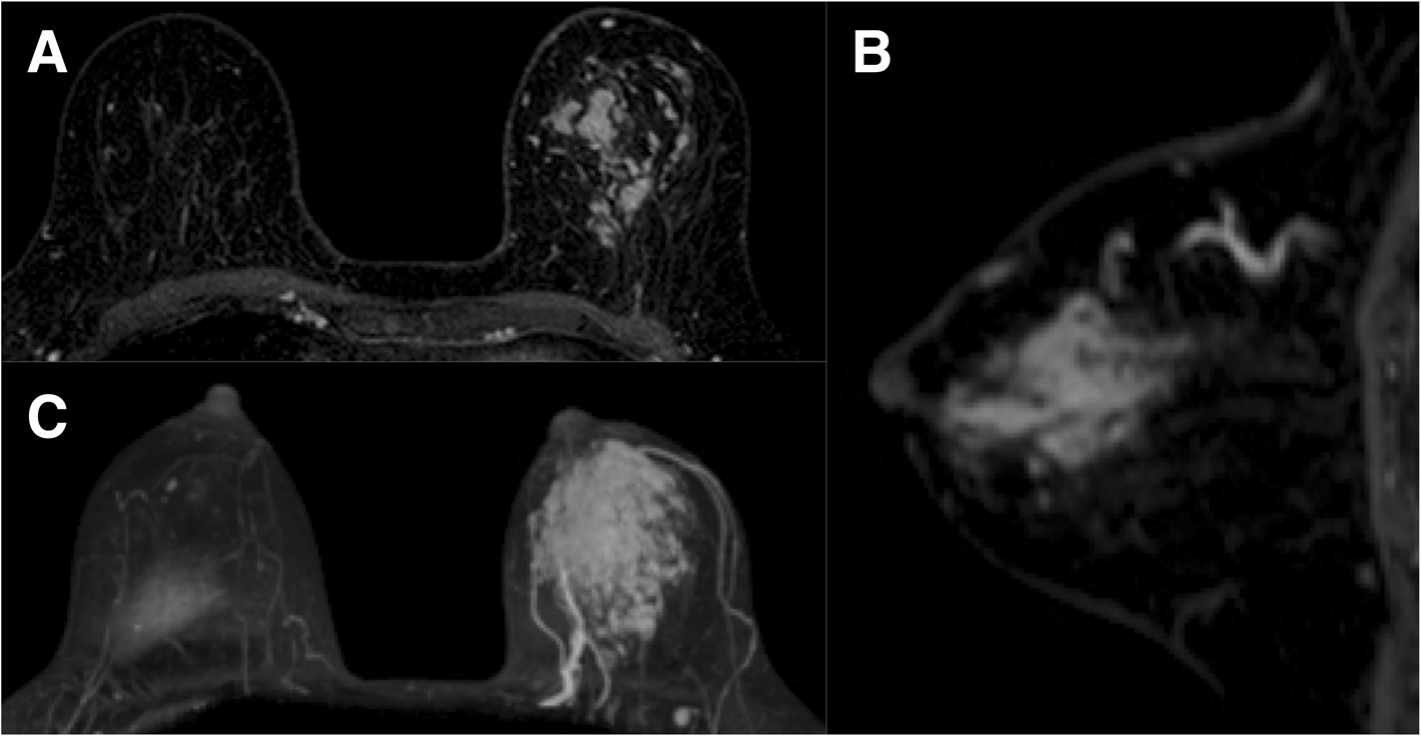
Findings from an enhanced magnetic resonance imaging scan of the breast. a, b Fat suppression. c MIP. Numerous punctate areas of contrast with dynamic washout were detected throughout the left breast. No invasion of the pectoral muscle, chest wall, or skin was observed
